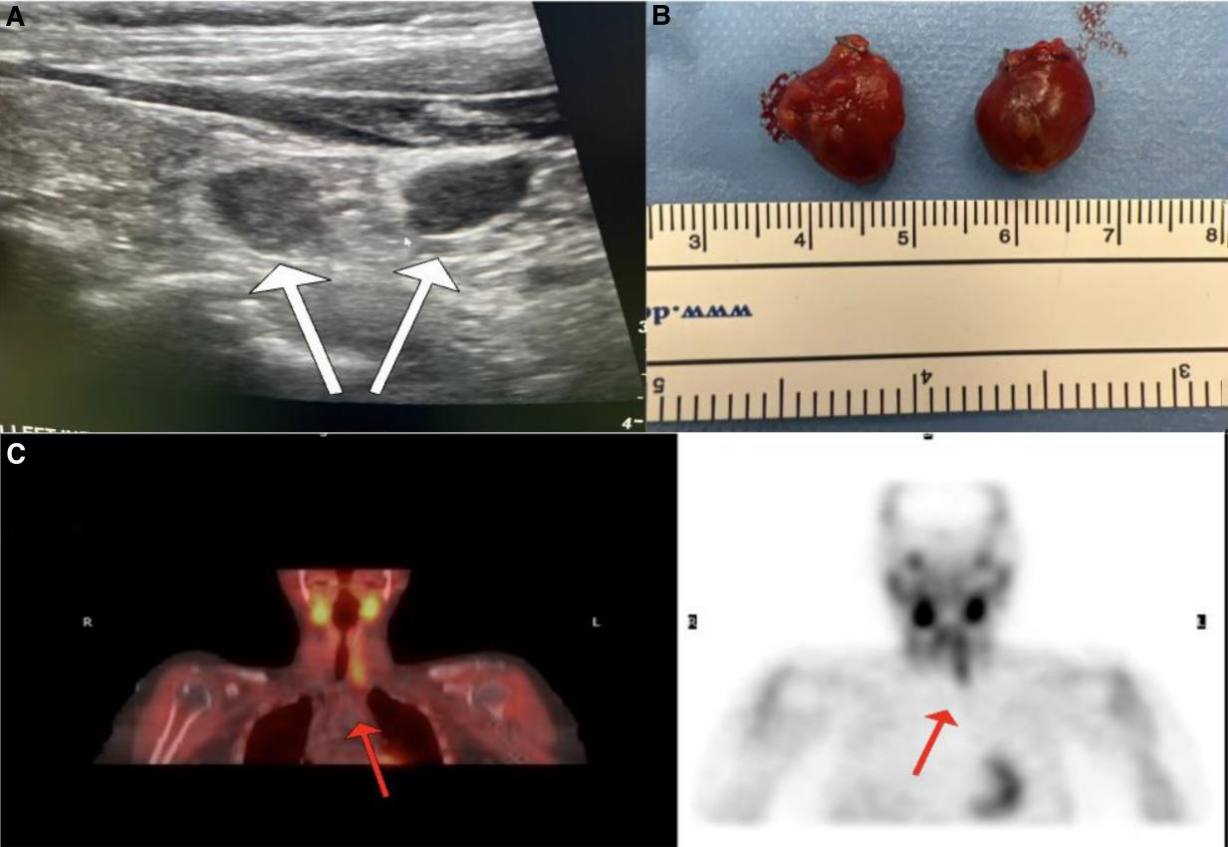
A, Ultrasound of the neck demonstrating 2 inferior parathyroid adenomas. B, The resected inferior parathyroid glands (gland 1: 1.6 × 1.1 × 0.6 cm and 0.5 g; gland 2: 1.5 × 1.1 × 0.4 cm and 0.57 g. C, Preoperative sestamibi scan demonstrating only one hyperfunctioning gland, left inferior parathyroid.
